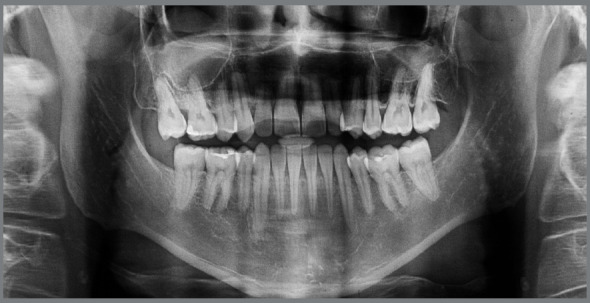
Panoramic radiograph 8 years after treatment.
